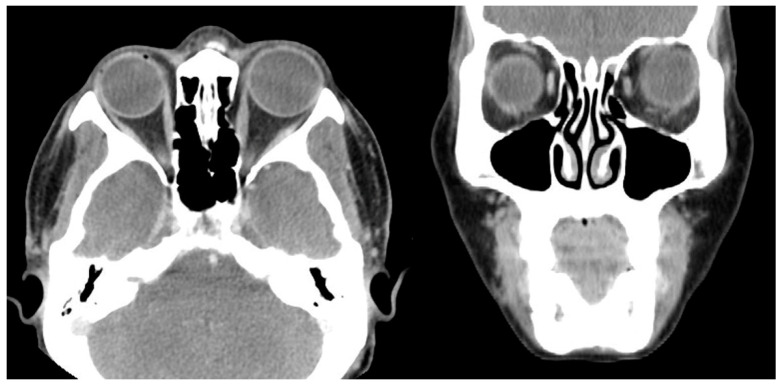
Postoperative CT image of Case 4. No definite abnormality is noted after left medial rectus muscle disinsertion.
